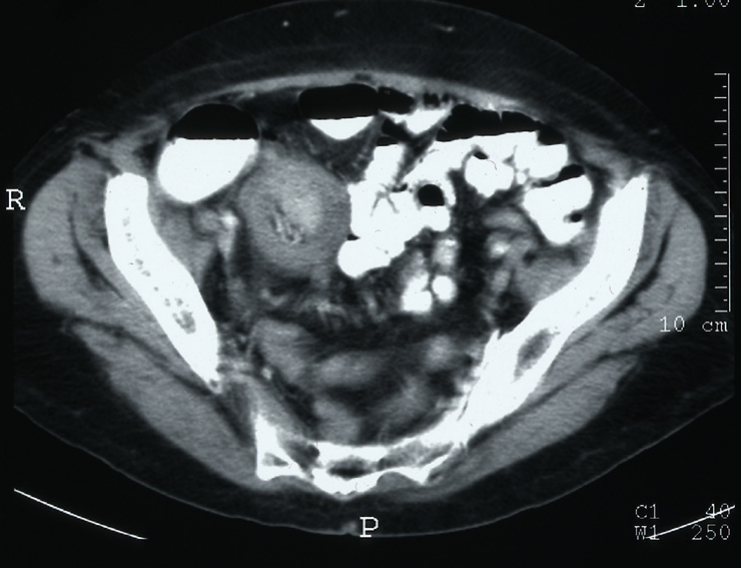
Contrast-enhanced computed tomography scan demonstrating a mass arising from the pelvis with a tubular appearance consistent with small bowel intussusception and mucosal oedema.
